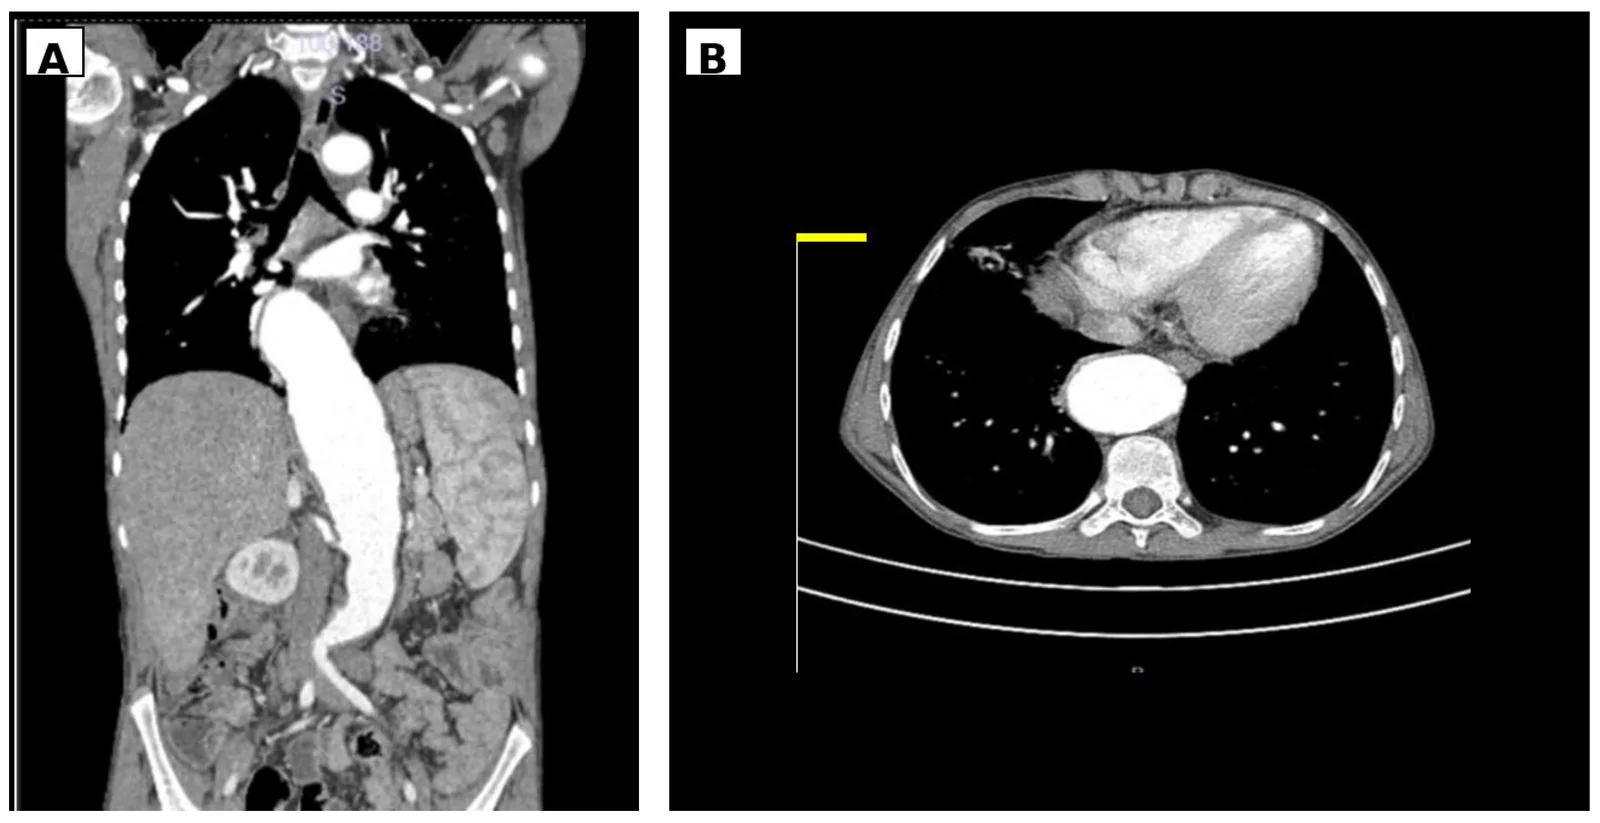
Giant aortic aneurysm in a patient with DOCK8 deficiency. (A,B) Coronal and axial thoracic CT angiography images showing a giant aortic aneurysm in one patient (F1.P1).
